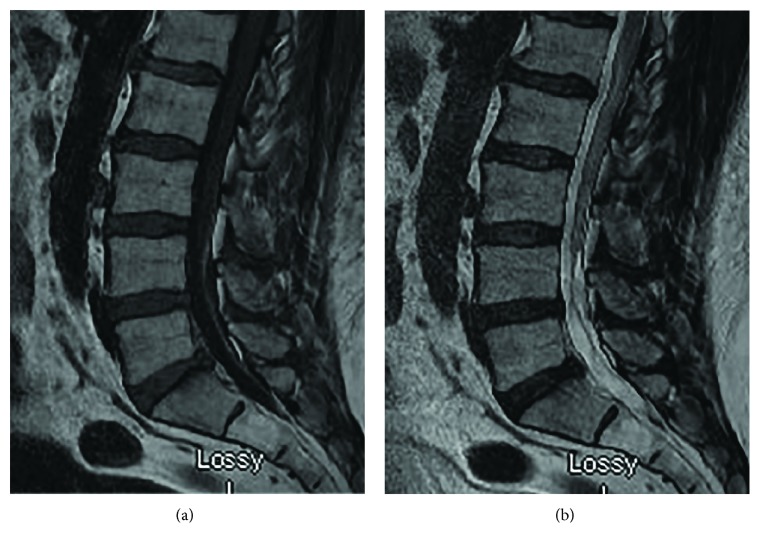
T1- and T2-weighted sagittal images of the lumbar spine revealing no acute processes. This study is from the initial workup in the emergency dept.
